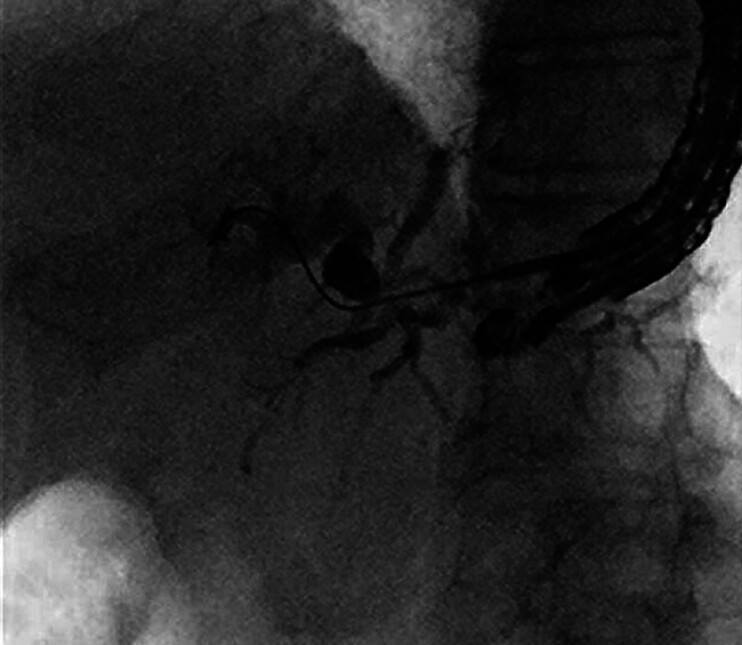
The guidewire perforated the bile duct and was misplaced outside the bile duct during endoscopic ultrasound-guided hepaticogastrostomy.
